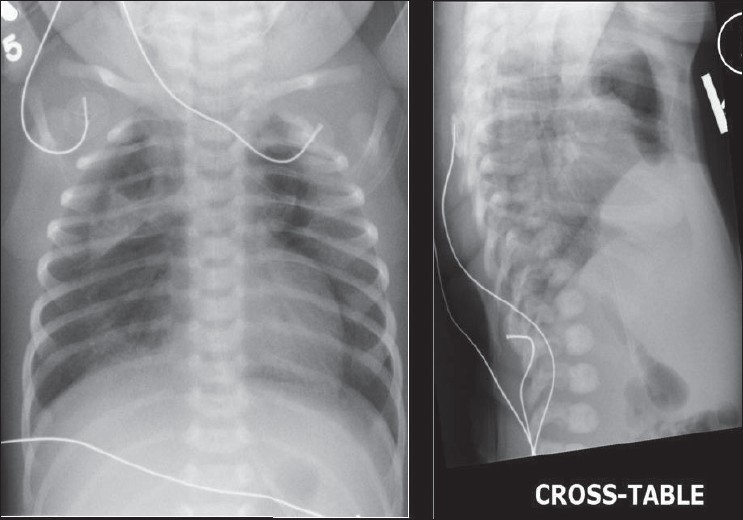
AP and cross-table chest radiograph on a 1-year-old child showing extensive pneumomediastinum; note that the air is extending to the root of the neck, differentiating it from a pneumopericardium)
